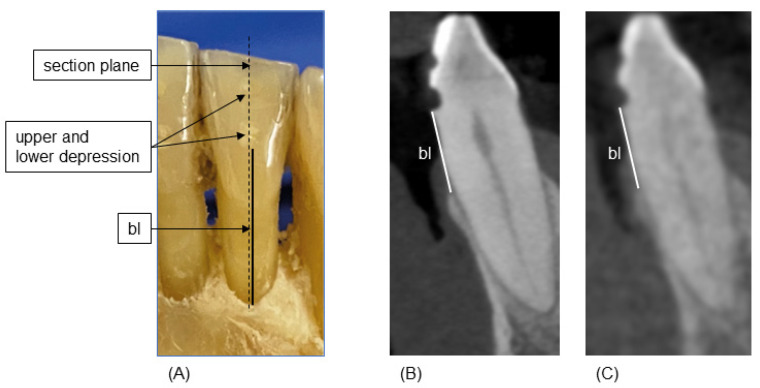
Measurement scheme. (A): reference measurements of buccal bone height (bl) from the most apical point of the lower depression to the most coronal point of the buccal bone (solid black line). (B,C): corresponding sagittal planes of HD-CBCT (B) and LD-CBCT (C). White lines indicate the bl.
